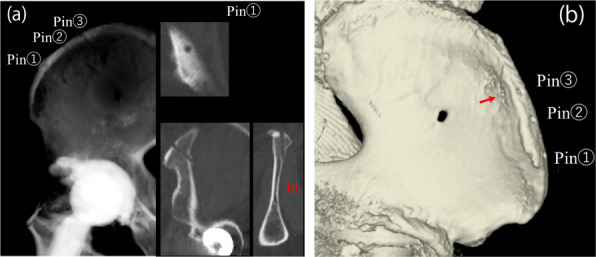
Postoperative CT 3D viewer analysis: a A digital projection and MPR views of the ilium; b A 3D pelvic model
